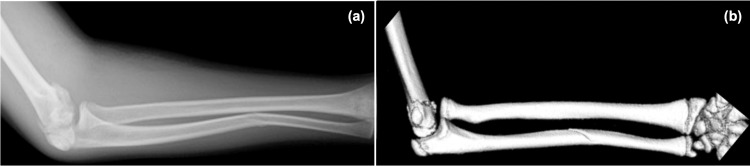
Forearm images on the day of injury (a) X-ray. (b) Computed tomography. Both showed an anterior dislocation of the radial head and an anterior convex to angulated deformity of the ulnar diaphysis.
